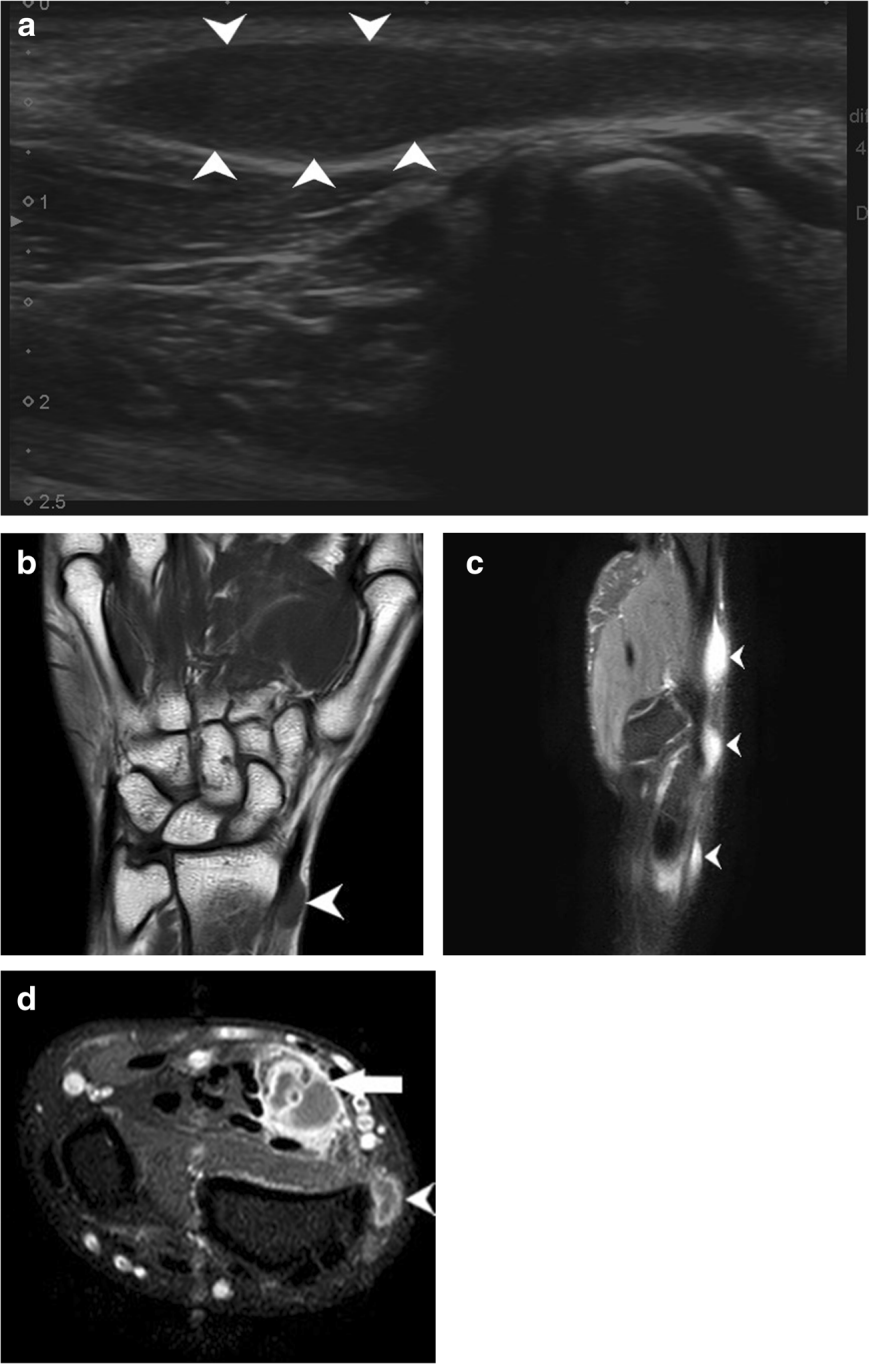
a Well-defined fusiform hypoechoic lesion (white arrowheads) is noted in continuity of the superficial branch of the radial nerve in the region of the anatomical snuffbox, just distal to the radial styloid. b T1-weighted coronal image shows another well-defined, T1 hypointense, fusiform lesion (white arrowhead) in continuity with the superficial branch of the radial nerve at the distal end of the radius. c PD fat-saturated sequence in the sagittal plane showing multiple PD hyperintense lesions (white arrowheads) along the superficial branch of the radial nerve. The lesions were hyperintense on T2 sequence also, showing internal fascicular areas suggestive of neural origin. d Axial post-contrast fat-saturated T1 image showing well-defined hypointense lesion surrounded by an enhancing capsule (arrowhead) in continuity with the superficial branch of the radial nerve. Another similar lesion (arrow) showing heterogenous contrast enhancement with hypointense areas within can be seen in continuity with the median nerve. This lesion was T2 and PD hyperintense with cystic degenerative changes on ultrasound and MRI. These MR findings fit the description of ancient schwannomas [33]. Ultrasound is a preliminary tool for identifying PNSTs, but MRI is the modality of choice as it helps in differentiating between neuromas and PNSTs
